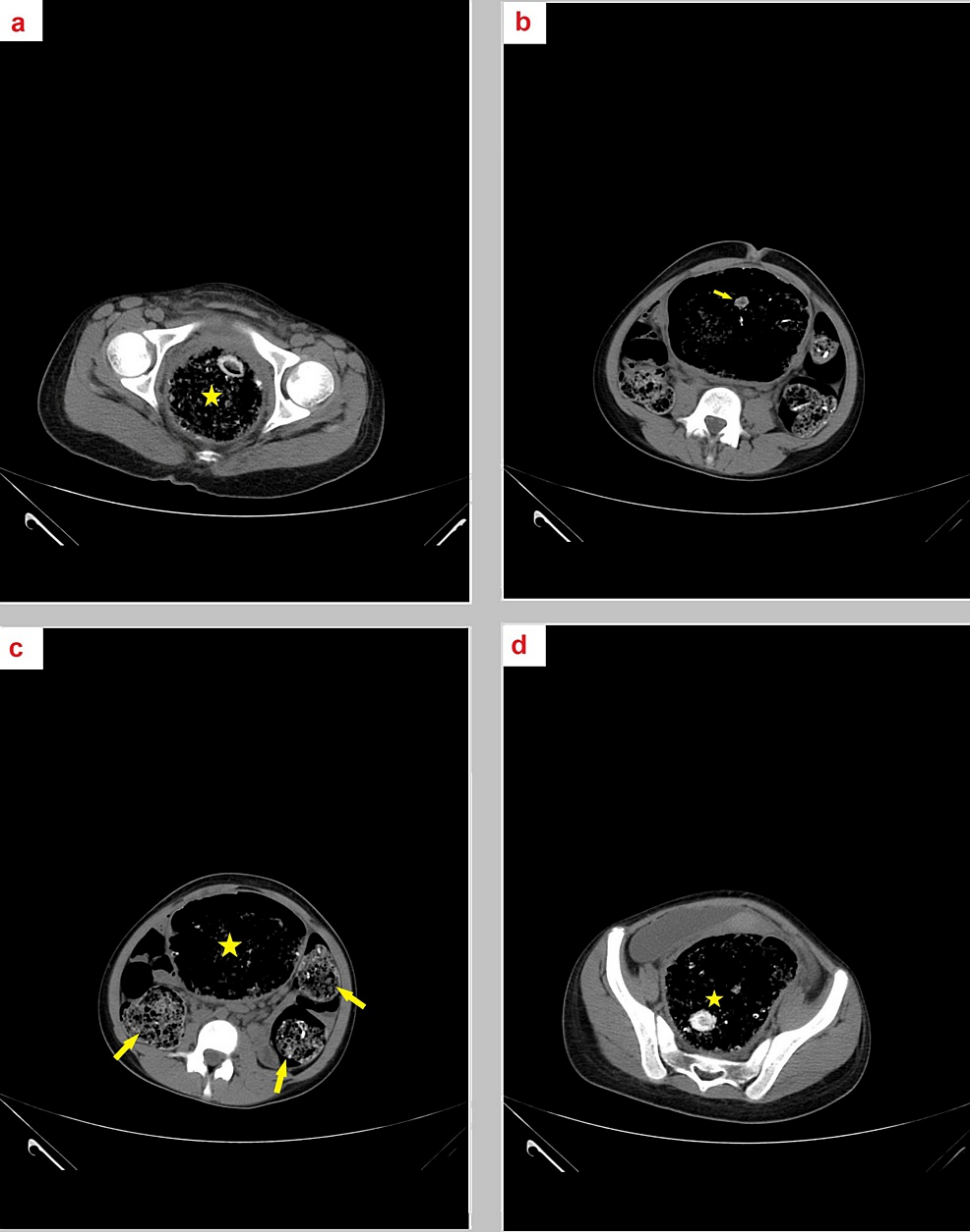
Computed tomography of the abdomen and pelvis (CTAP) displays a dilated large bowel loop with bizarre material present with a severely dilated colon, causing a mass effect on the small bowel loop and the urinary bladder (a-d)
